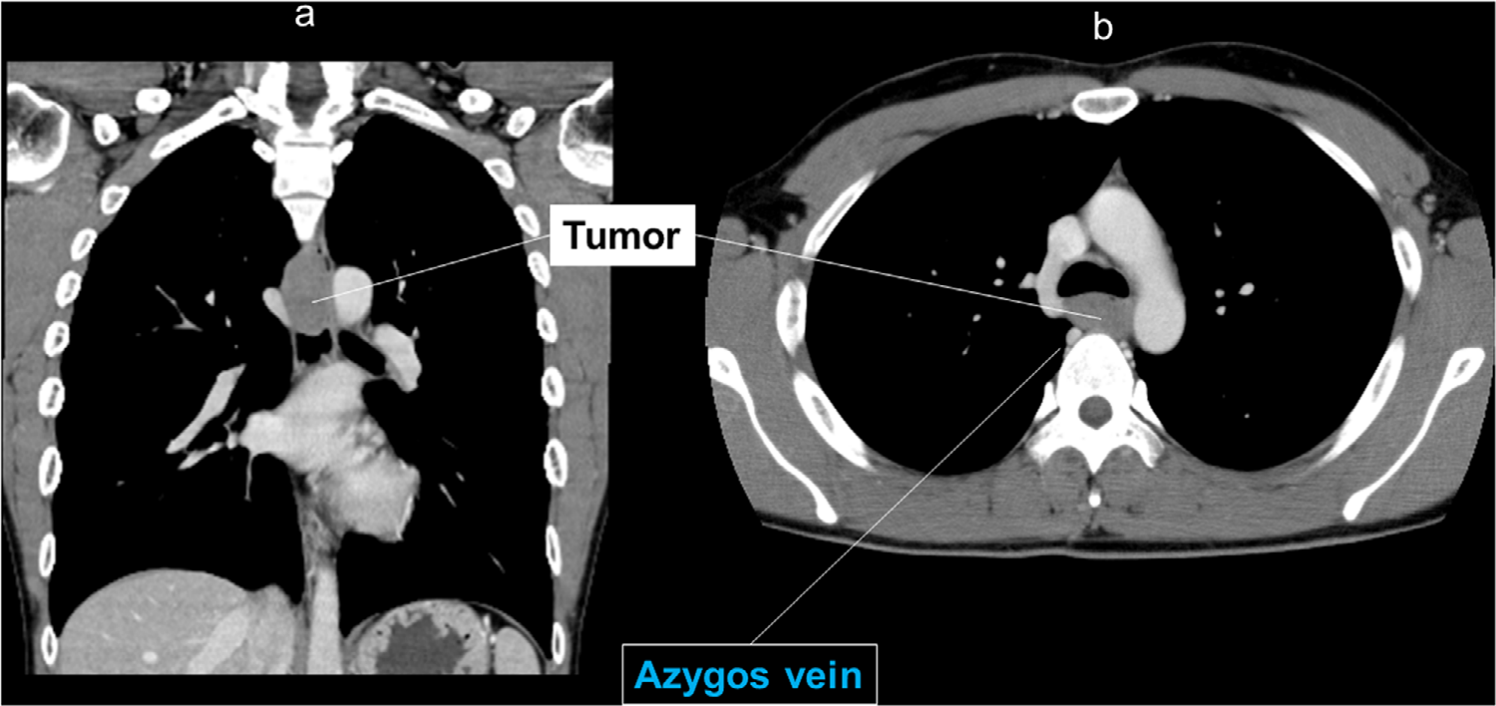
(a) Computed tomography showed a uniform low‐density mass in the mediastinum. Tumor size was 40 × 25 mm. (b) CT showed the azygos vein posterior to the tumor
